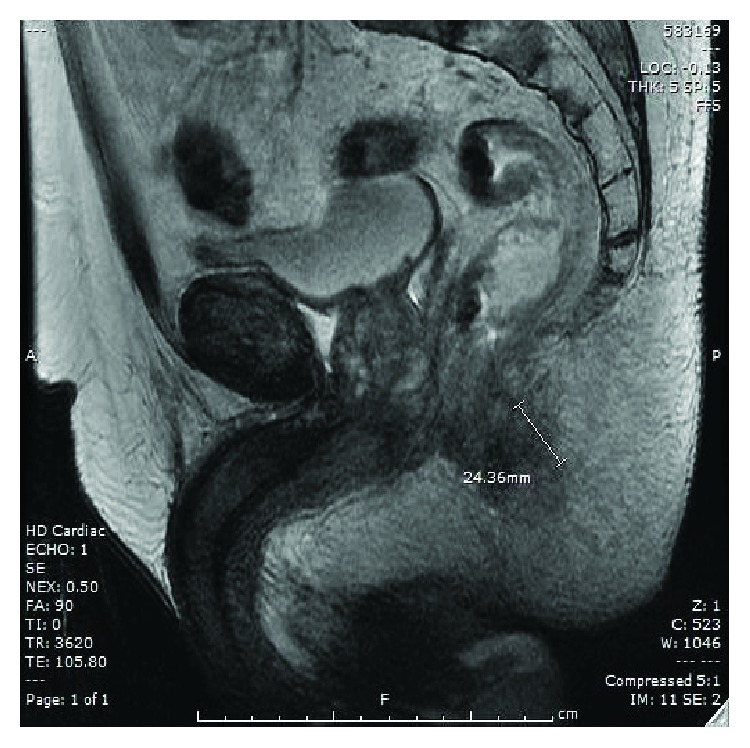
Lateral view of pelvic MRI showing inflammatory changes involving mucosa of the anus, rectum, and internal sphincter.
